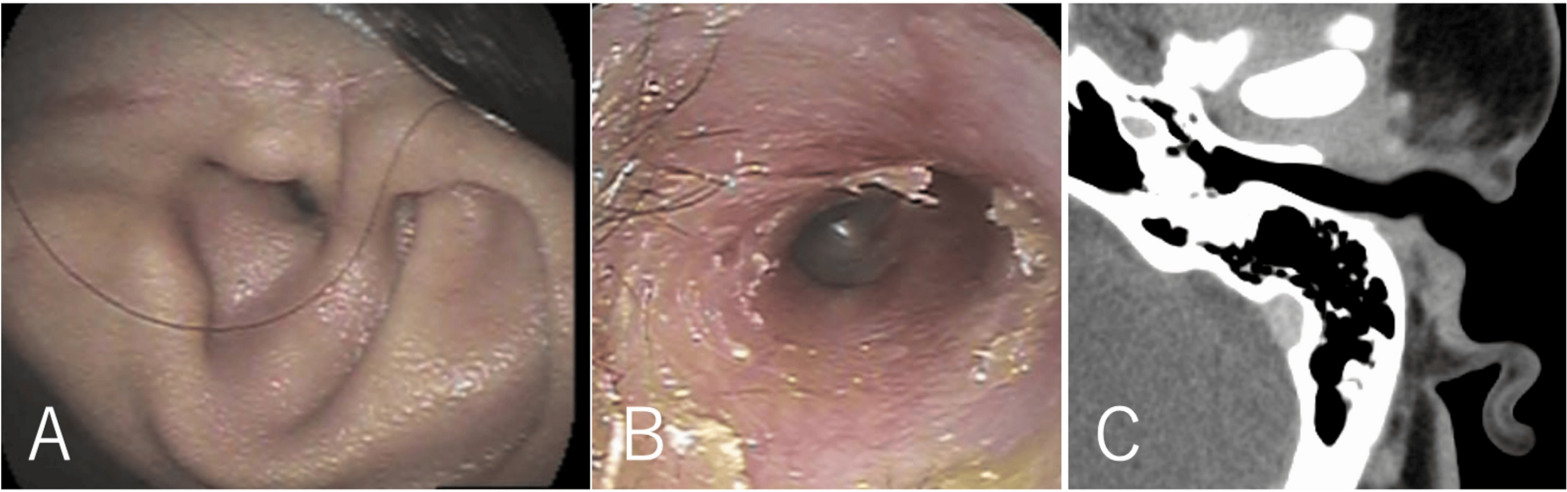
Postoperative findings Postoperative photographs of the left auricle (A) and external auditory canal (B). (C) Contrast-enhanced CT image taken five months postoperatively. The tumor has disappeared, and the external auditory canal is well-epithelized, with the tympanic membrane visible. Contrast-enhanced CT showed no sign of recurrence.
